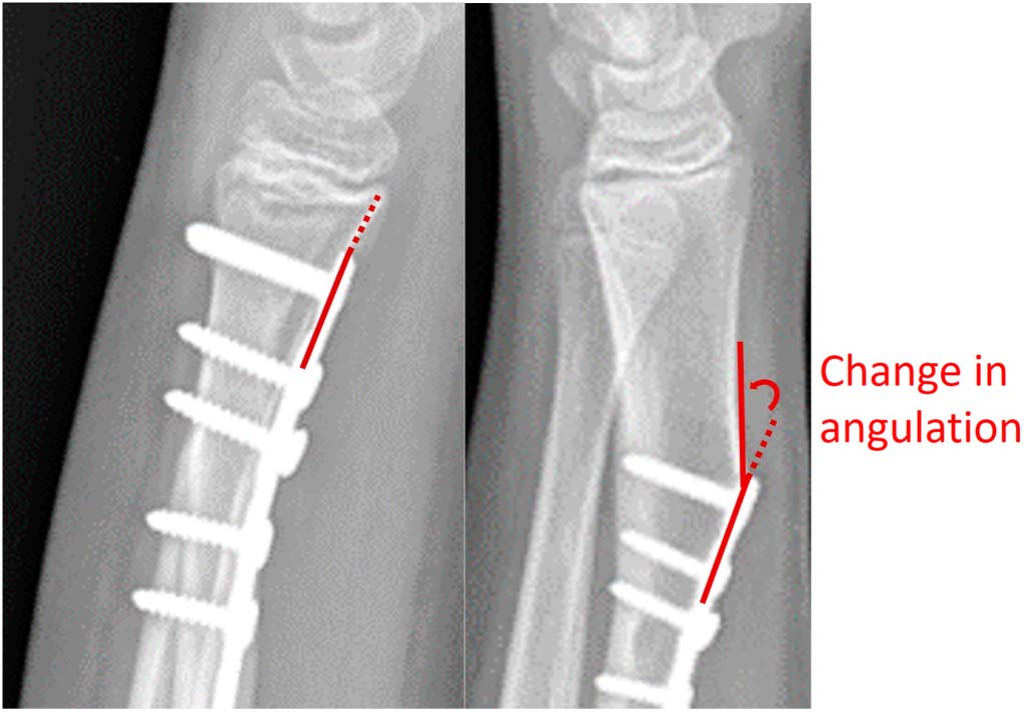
Lateral radiograph of the wrist demonstrating how angular deformity in the radius was measured.
